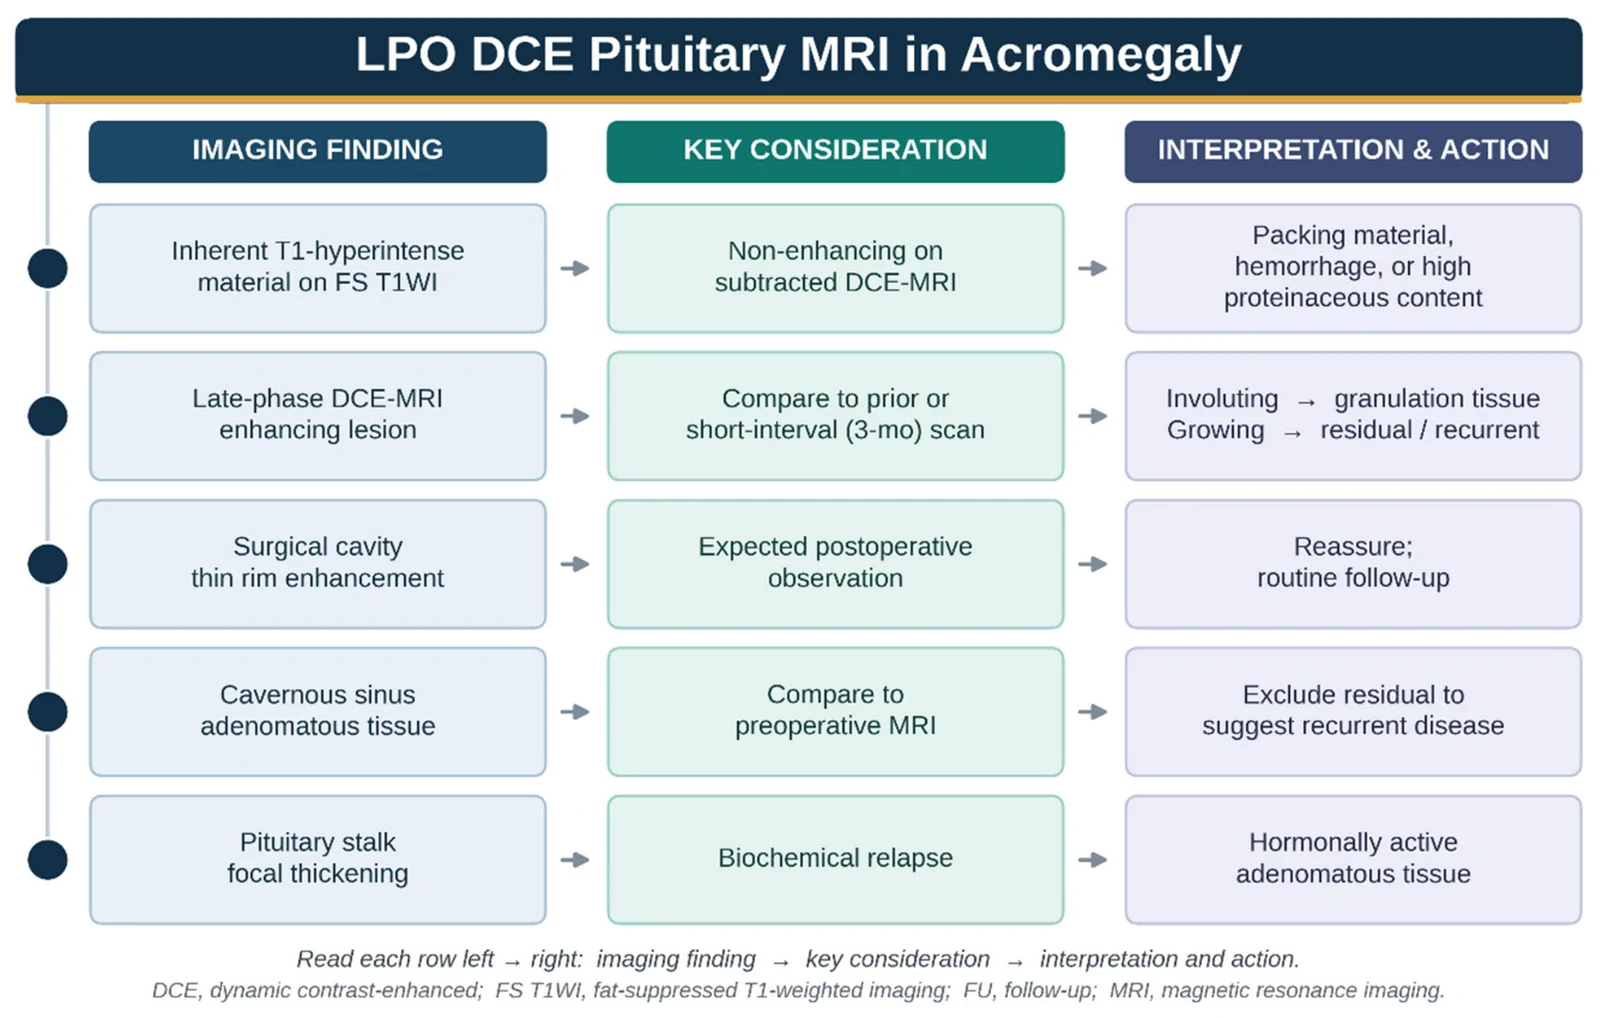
Late Postoperative Pituitary MRI Patterns (following transsphenoidal surgery for acromegaly).
